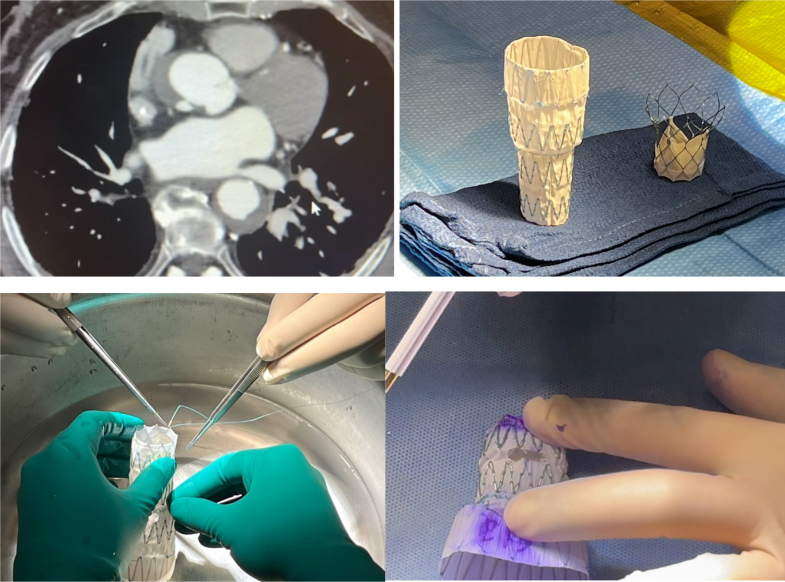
Preoperative computed tomography angiography scan. Thoracic endovascular graft and self-expanding transcatheter valve are hand-sewn together. Fenestration is created in each cusp (3 total).
